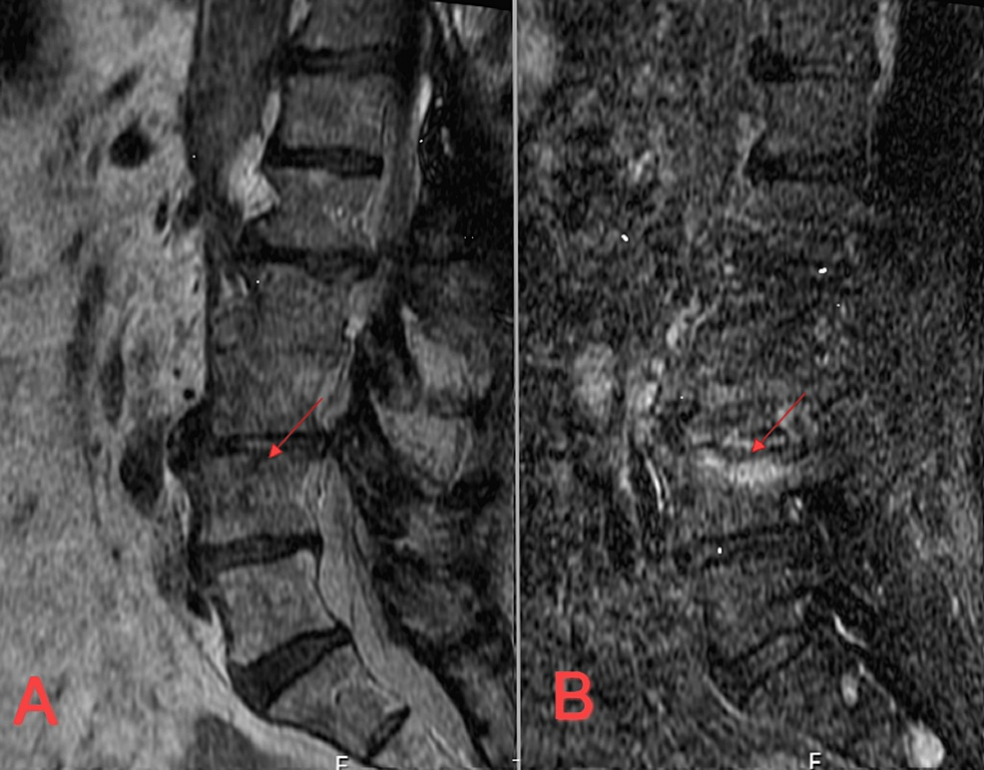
A) a sagittal T2-weighted image of the lumbar spine and the arrow indicates site of osteomyelitis at the level of L3-L4. B) is a corresponding STIR sequence noting ongoing boney infection. STIR: short inversion time inversion recovery
